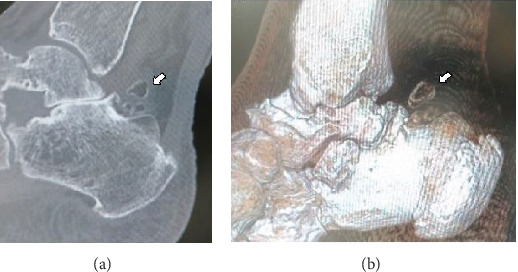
Computed tomography scans showing homogeneous calcifications with typical ring-and-arc chondroid mineralization in the posterior ankle (a) on a plain sagittal view (arrow) and (b) on a three-dimensional image view (arrow).
